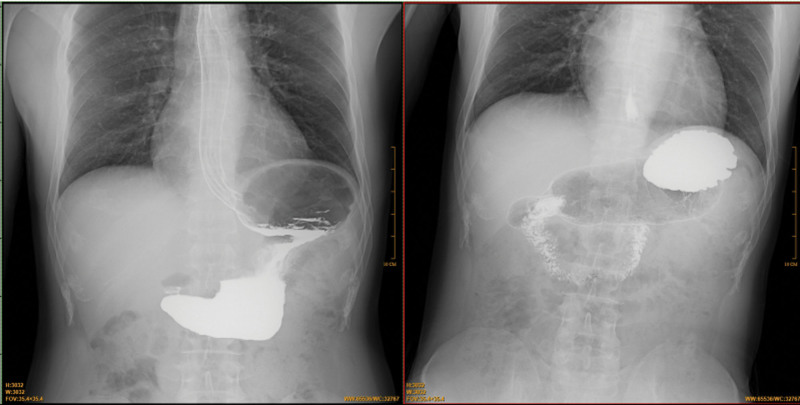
The digital gastrointestinal radiography (GI) showed the gastric sharp angle sign and the mucosal folds were irregular occurred at the lesser curvature of stomach.
